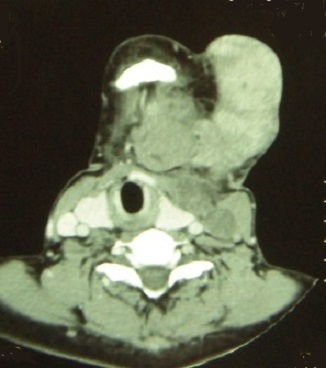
An axial CT scan view showing tethered skin and left jugulodigastric lymph node enlargement with central necrosis
